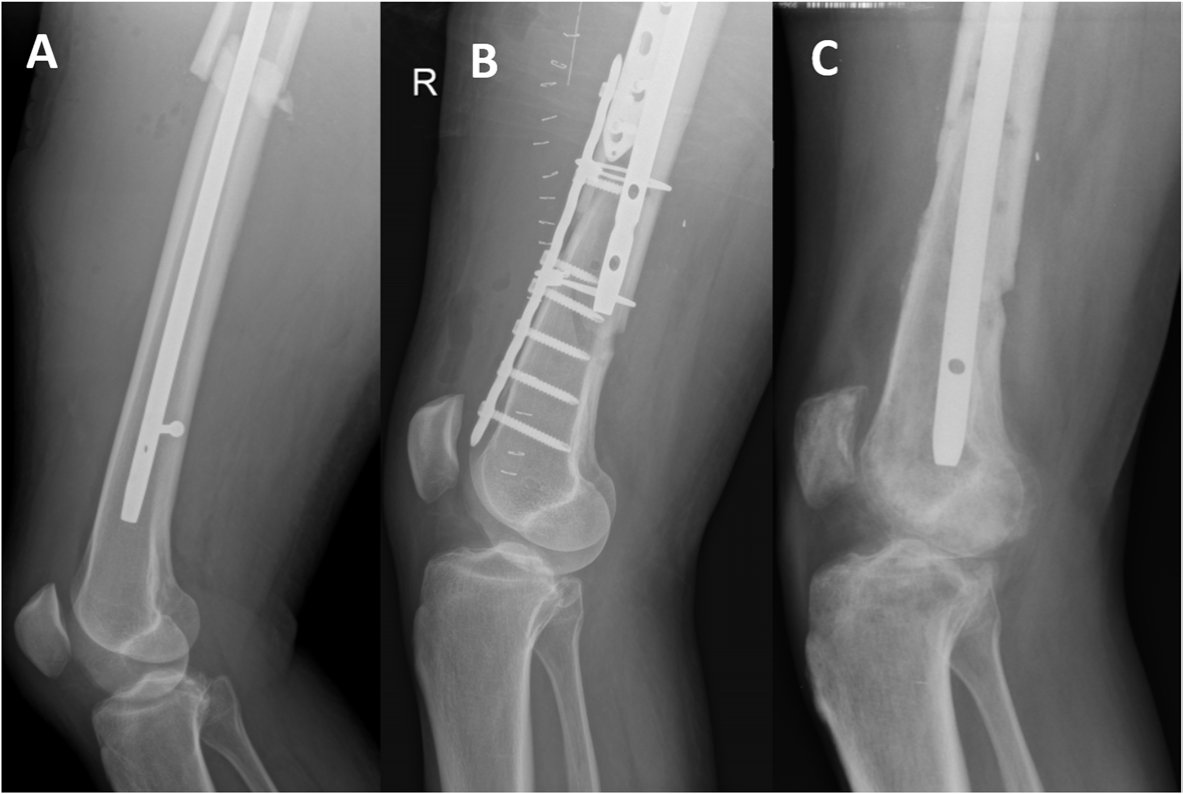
A X-ray seven days after initial open reduction and intramedullary nailing (nail Gamma Long, Medgal), June 2017. Destabilization of the fixation and migration of the femoral fragments can be seen. Anterior tibial subluxation is not present yet. B Postoperative X-ray after first reoperation due to destabilization of initial fixation, June 2017. Anterior tibial subluxation can be seen. C Postoperative X-ray after second reoperation due to nonunion, April 2018. Anterior tibial subluxation and limited knee flexion is still present despite manipulation under anesthesia
